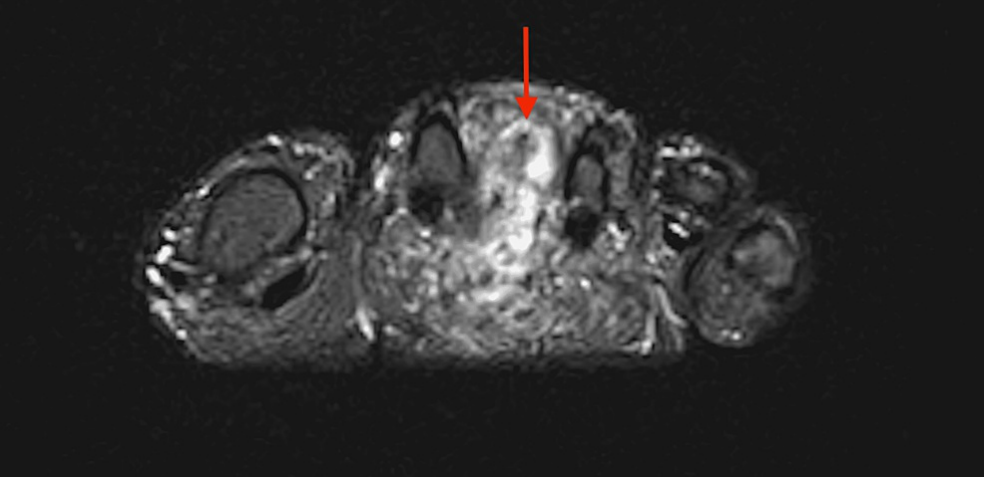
Magnetic resonance imaging displaying a soft-tissue mass with high signal intensity between the second and third metatarsal in a short tau inversion recovery (STIR) imaging sequence
